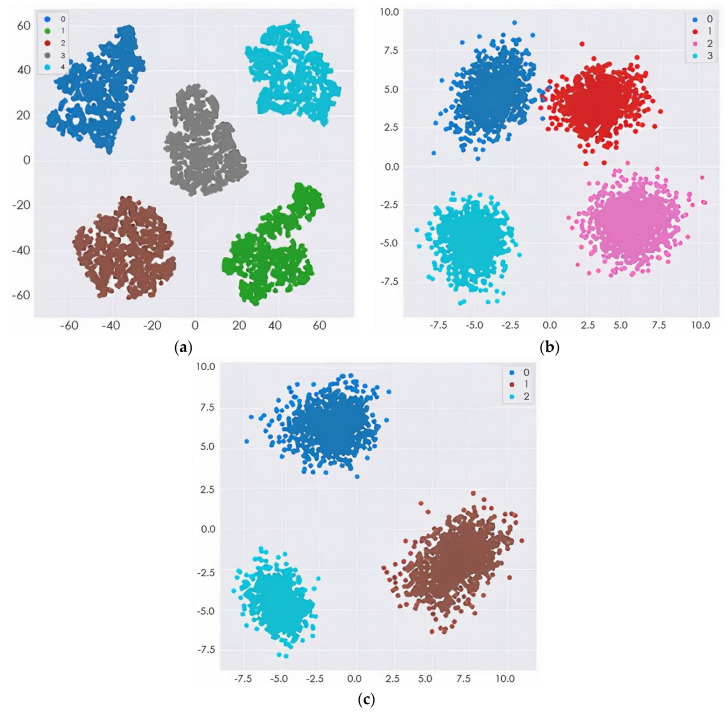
The t-SNE projection visualization of several MRI features: (a) 5-way multiclass classification; (b) 4-way multiclass classification; (c) 3-way multiclass classification.
